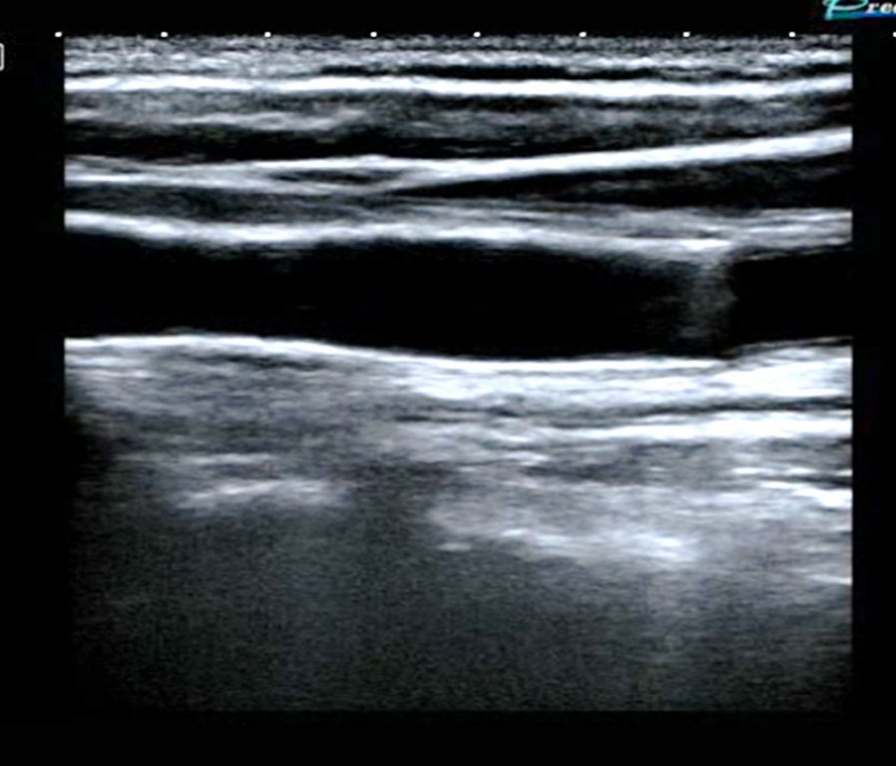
Ultrasound of carotid after stenting: Longitudinal view
